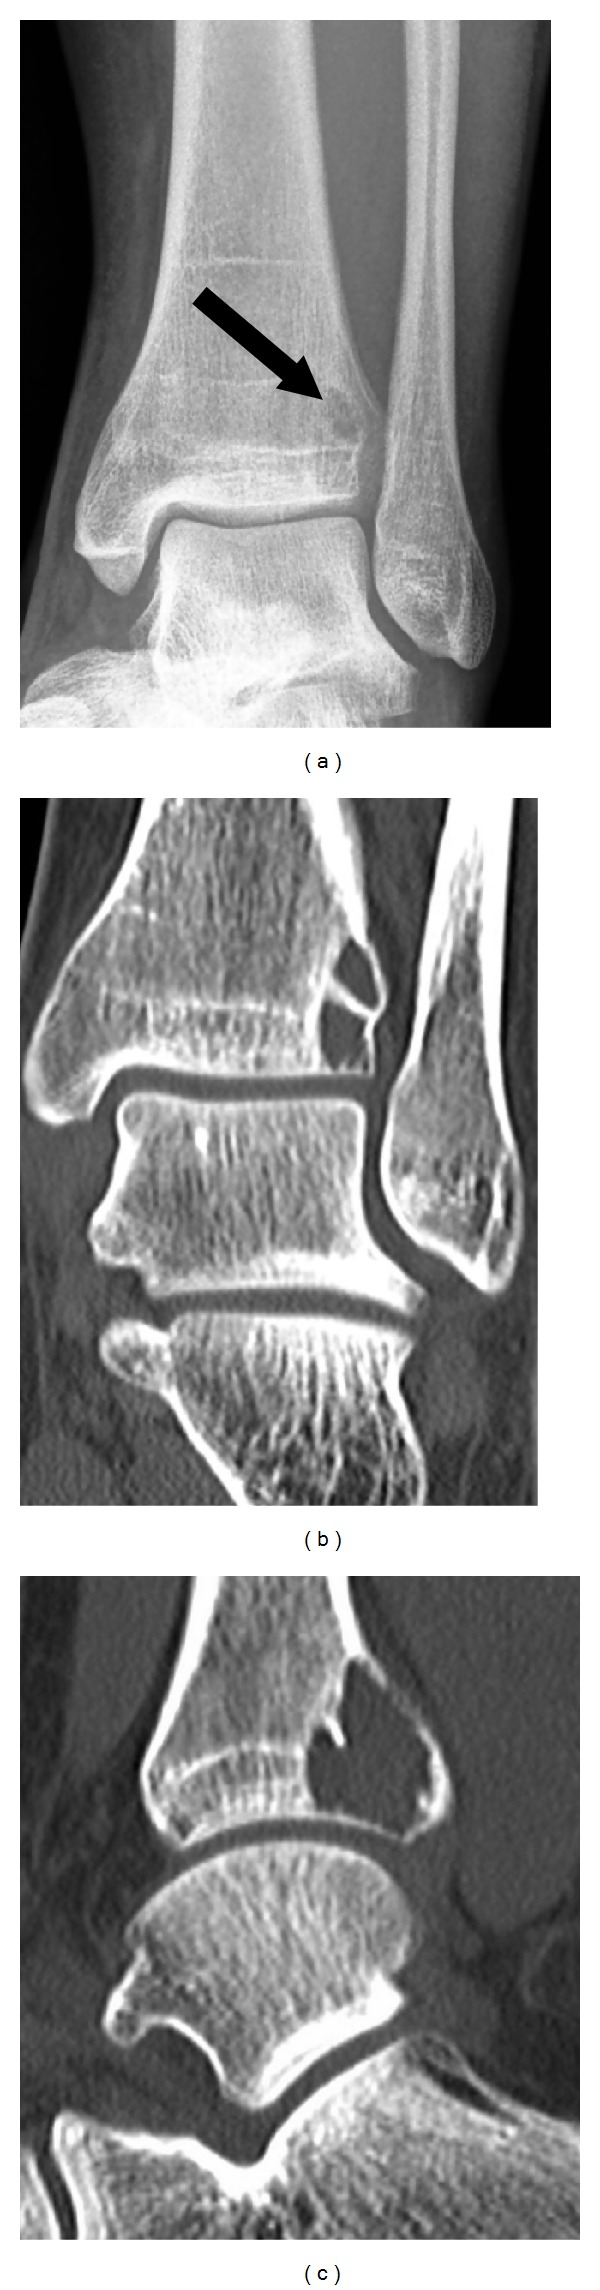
Conventional radiography and computed tomography of the left distal tibia: (a) plain film (AP view) presents the lytic lesion (arrow) of the left lateral distal tibia, and (b) coronal and (c) sagittal CT sections can recognize the exact extension of the subchondral distal region of the dorsolateral left tibia.
